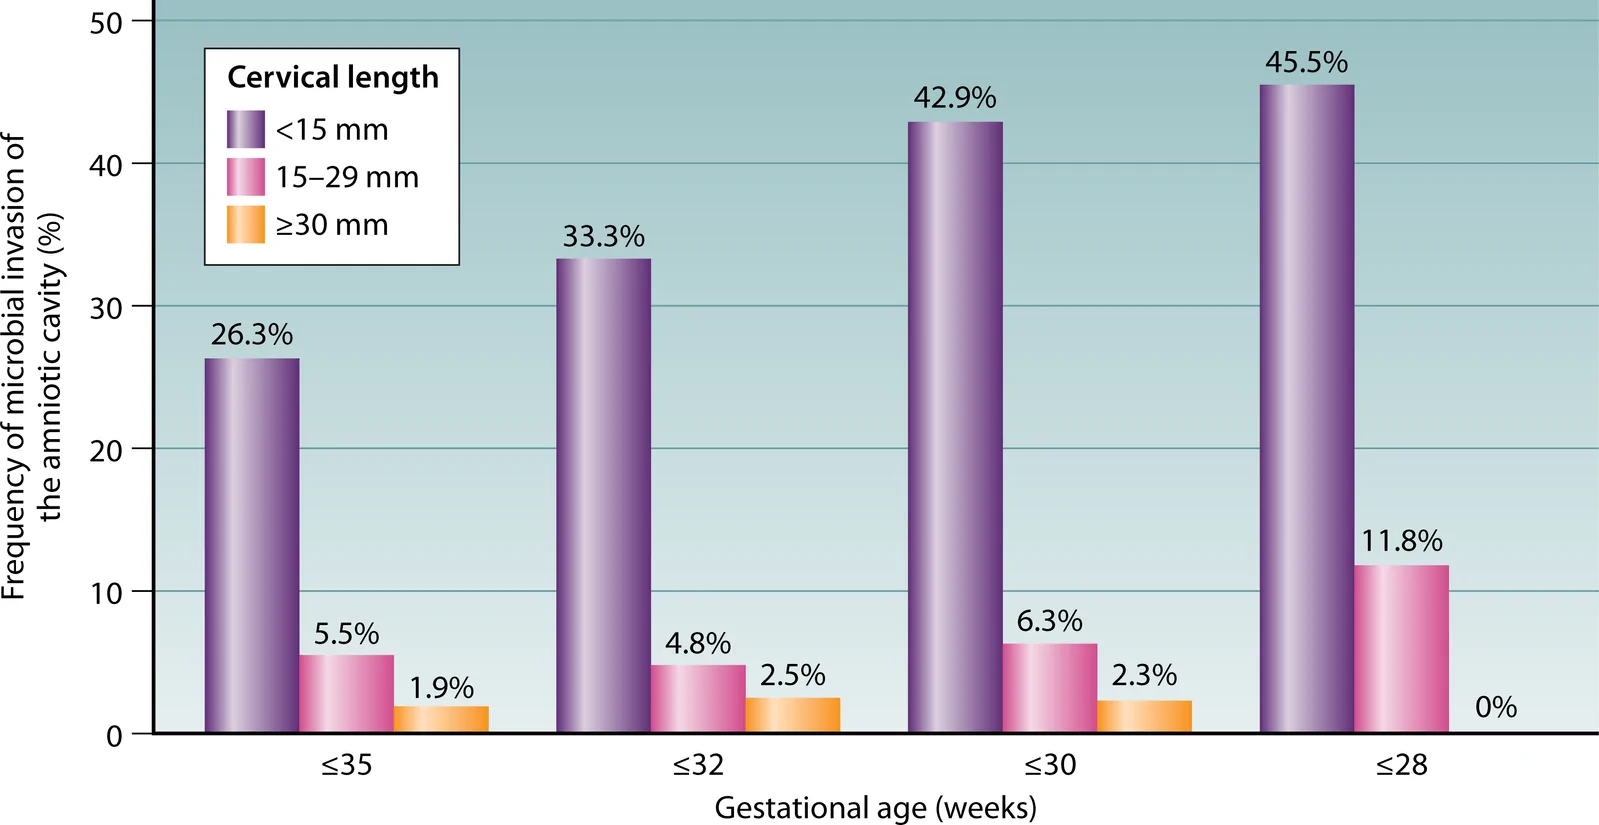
The relationship between the frequencies of microbial invasion in the amniotic cavity and ultrasonographic cervical length. Data from reference 102.
